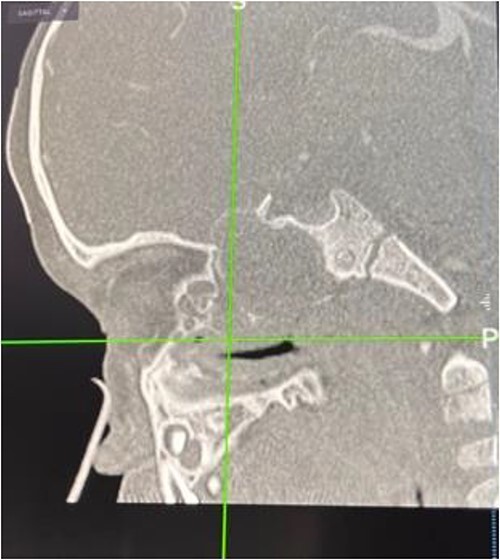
Skull base erosion on CT navigation.
